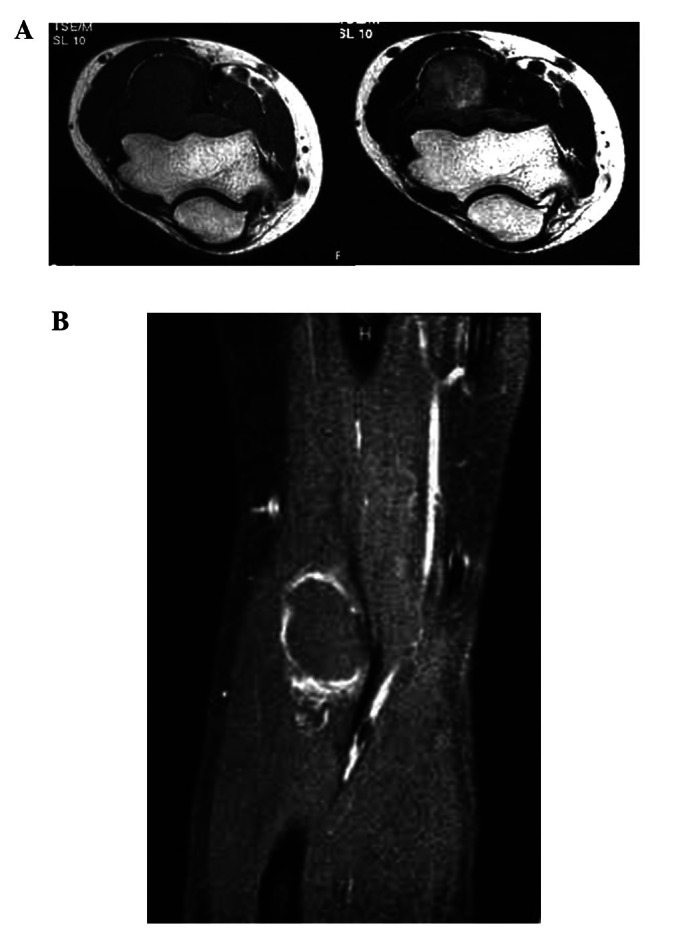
(A) Magnetic resonance image showing the tumor located between the brachioradialis and brachialis. The signal intensity of the tumor was isointense to muscle on T1-weighted images (left) and hyper- or isointense to muscle on T2-weighted images (right). (B) On gadolinium-enhanced magnetic resonance images, the margin of the tumor was well-contrasted.
